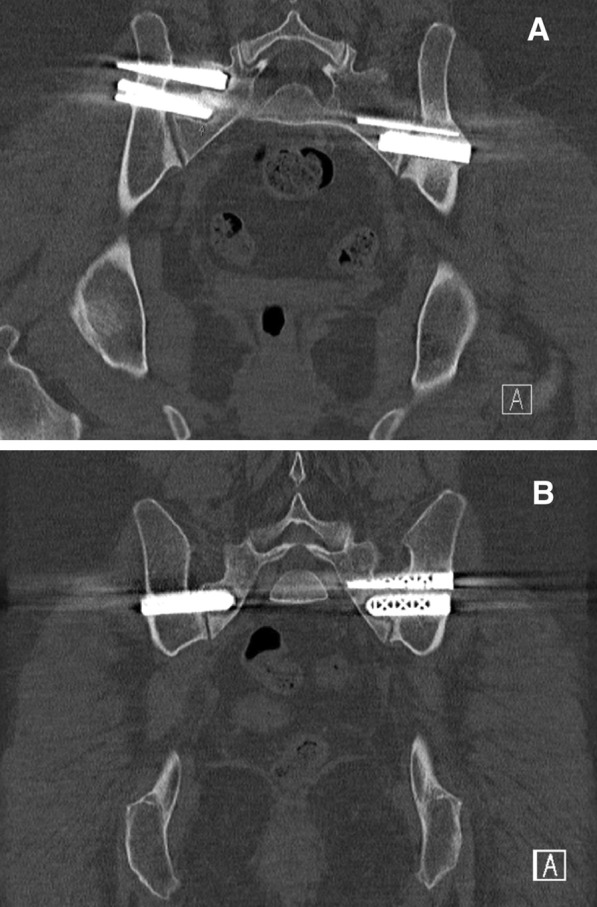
Two cases of implant loosening on pelvic CT scan
